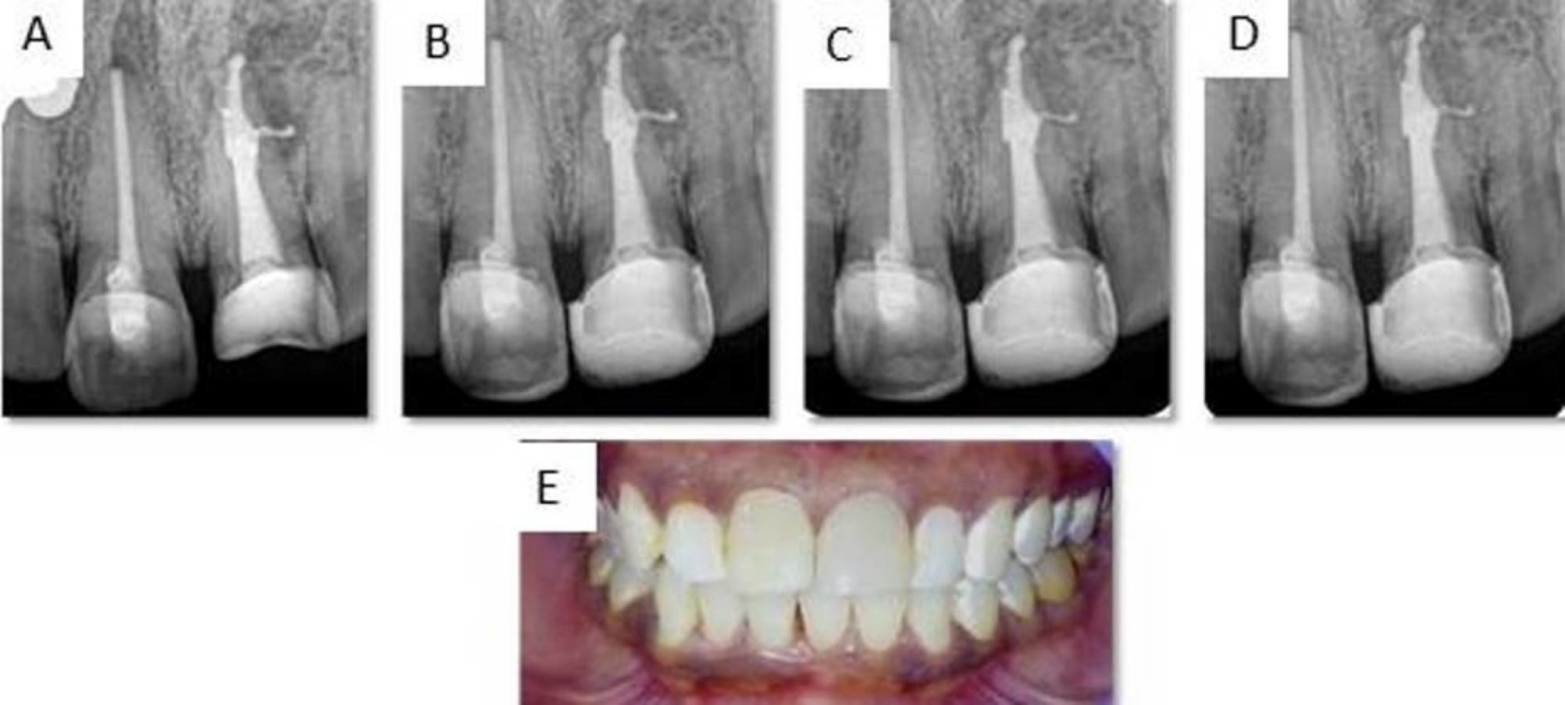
Postoperative radiograph and photograph of tooth 11 and 21. (A) Obturation. (B) Six-month follow-up. (C) 12-month follow-up. (D) 18-month follow-up. (E) Post operative photograph.
